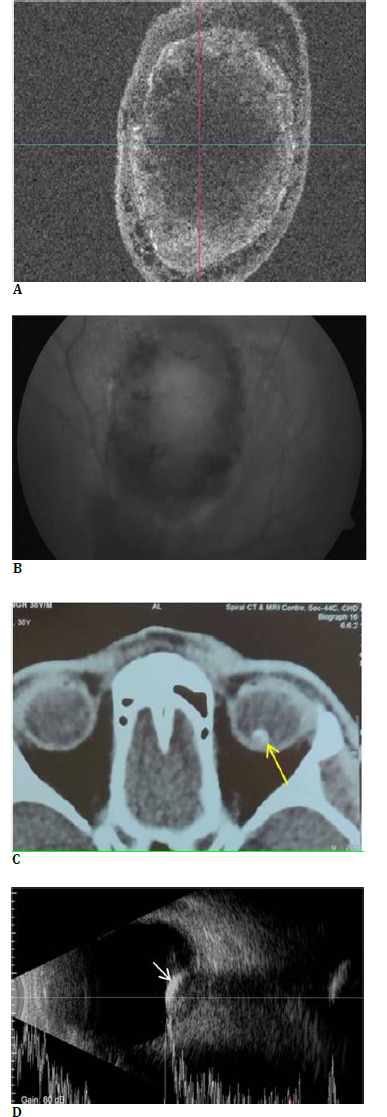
Optical coherence tomography (OCT) enface images (A) of the choroidal mass show an oval lesion with a hyperreflective rim and surrounding hyporeflective cystic spaces, giving an eggshell-like appearance. Fundus autofluorescence (B) shows central iso-autofluorescence with surrounding hypo-autofluorescence. NCCT scan of the left orbit (C) shows a hyperdense rim of calcification (yellow arrow) around the affected choroid. Ultrasonography B/A scan of left globe shows (D) a dome-shaped hyperechoic lesion (white arrow) with surface hyperreflectivity and posterior backshadowing suggestive of calcification
